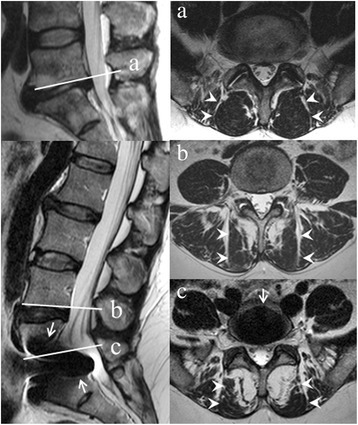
Grading of fat in the multifidus muscles on magnetic resonance imaging. Multifidus muscles (right, *arrowheads*) on axial T2-weighted images located as marked on sagittal T2-weighted images (left, *lines*) contain fat grade 0 at L5/S1 in one patient (a) and grade 1 at L4/L5 (b) and grade 2 at L5/S1 (c) in a different patient, whose disc prosthesis causes artefacts (*arrows*) that do not affect the grading. Grade 0: 0 or < 20% of total muscle cross-section (left plus right side) contains fat; grade 1: 20–50% of cross-section contains fat; grade 2: >50% of cross-section contains fat
